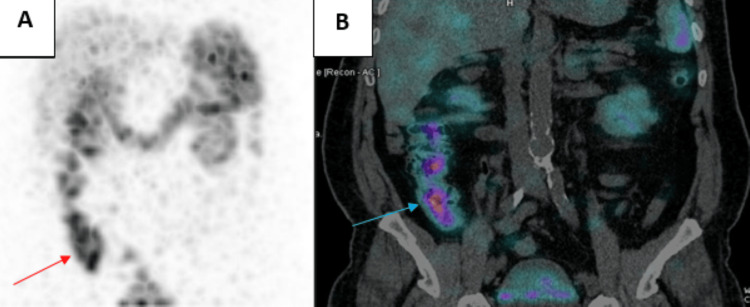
An octreoscan of our patient focused on the lesion located in the cecal base, combined with single-photon emission computed tomography coupled with computed tomography. A: Octreoscan showing intense tracer uptake at the base of the cecum (red arrow); B : Single-photon emission computed tomography coupled with computed tomography showing the intense uptake in the cecal base (blue arrow)
